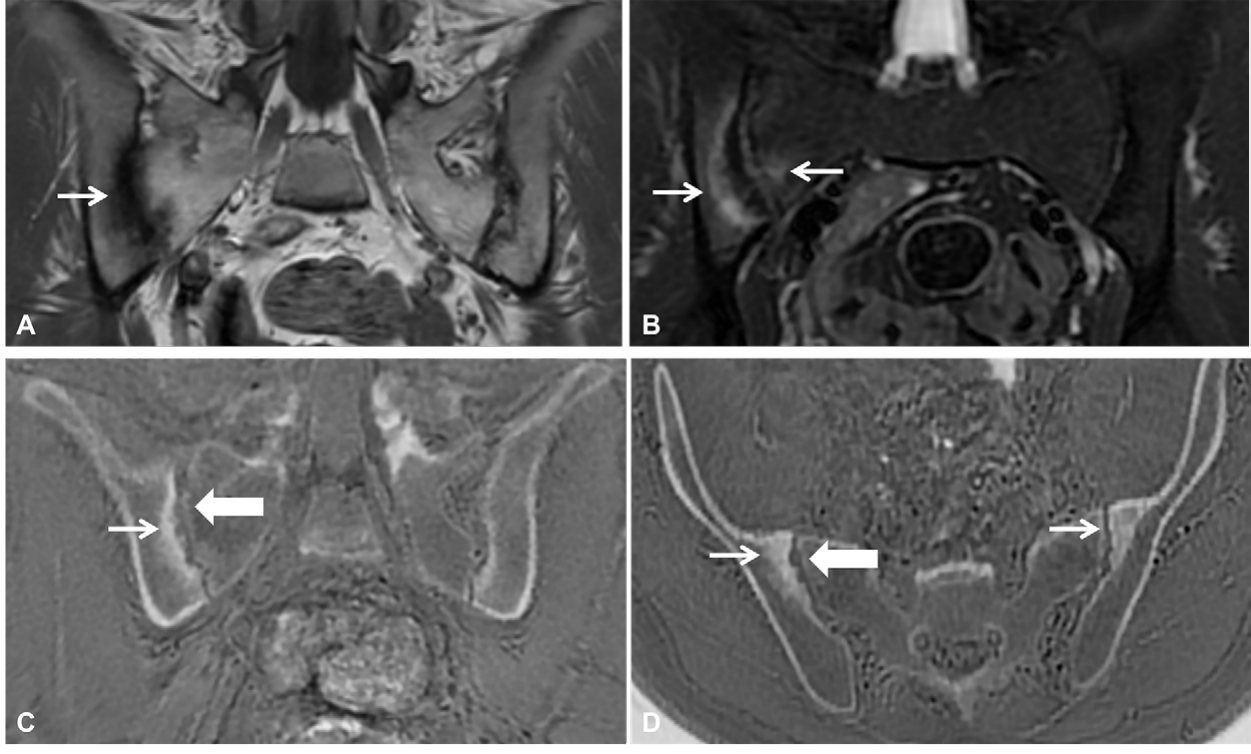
MRI of the pelvis in a 32-year-old male runner presenting with low back and buttock pain radiating to the right leg. (A) Oblique coronal T1-weighted image demonstrates irregular and blurred articular margins of the right sacroiliac joint with subchondral low signal intensity (arrow). (B) Oblique coronal T2 fat-suppressed image demonstrates extensive bone marrow edema adjacent to the right sacroiliac joint. (C, D) Oblique coronal and axial ZTE images show sclerosis (arrow) and erosions (bold arrow), orienting the diagnosis to inflammatory sacroiliitis, which was proven after rheumatologic investigation.
